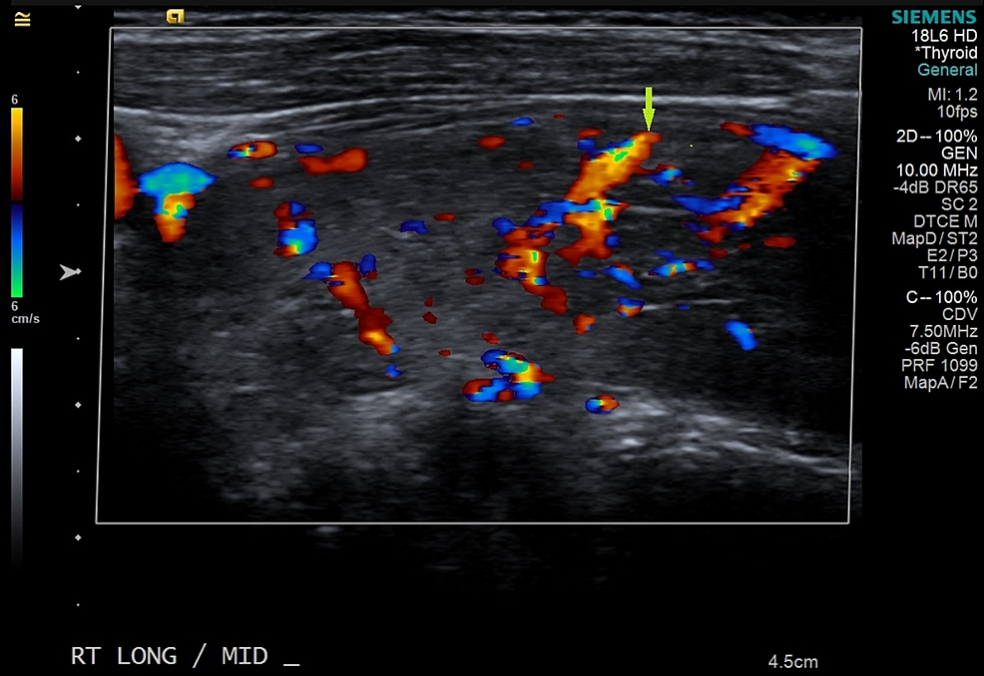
Ultrasound revealed enlarged heterogeneous thyroid gland with increased vascularity
